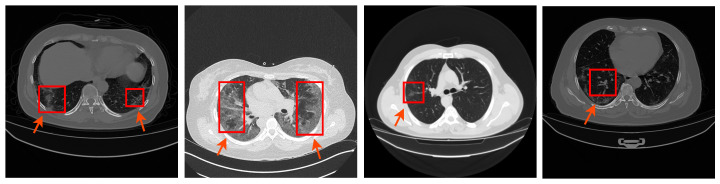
Four examples of COVID-19 existing in chest CT images (COVID-19 infection is highlighted by red boxes).
